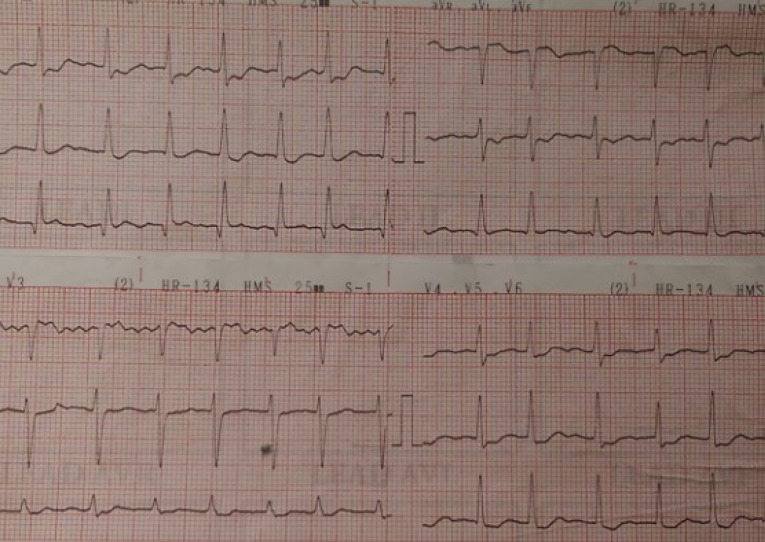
Echocardiography was repeated and showed normal EF. No pericardial effusion but there was a mass compressing RCA ostium.
